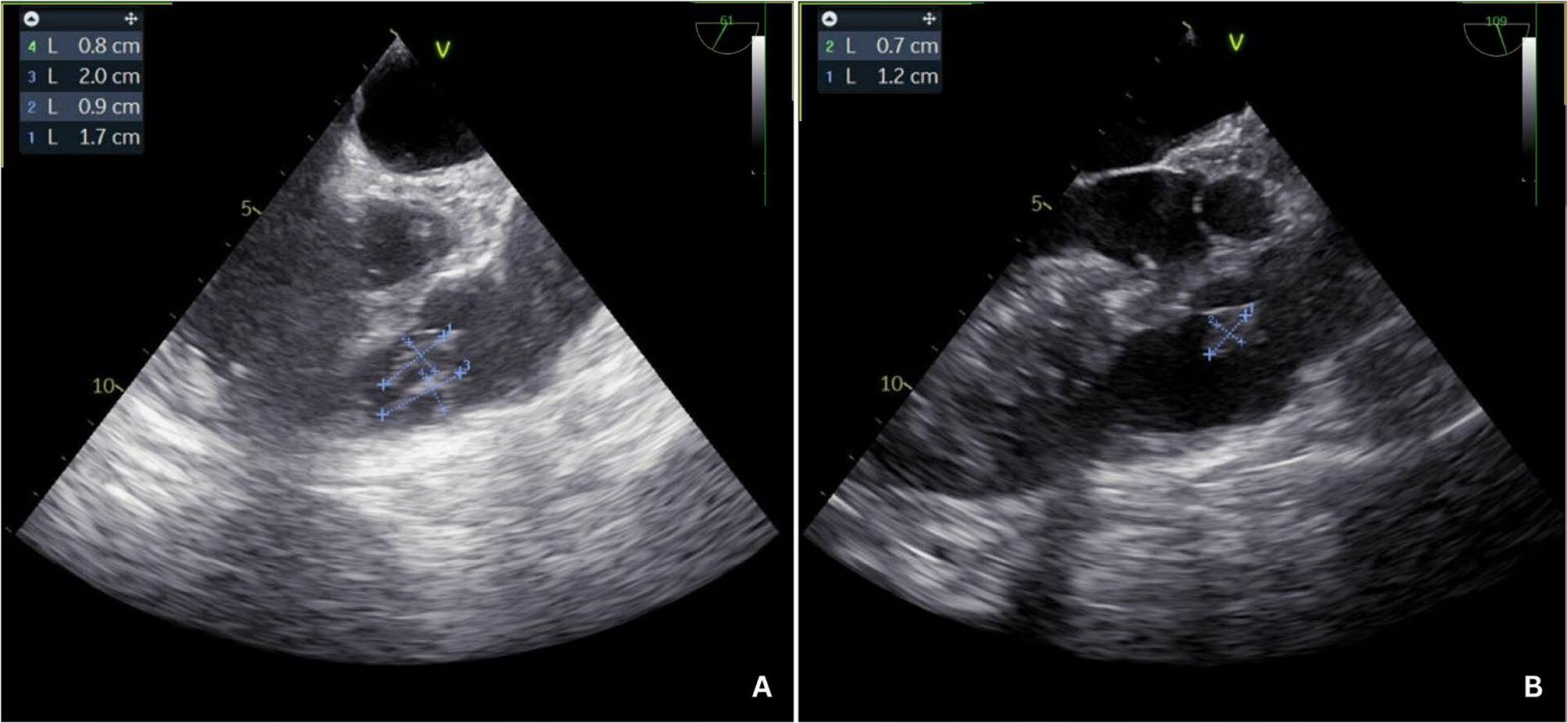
Transesophageal echocardiogram (TEE). A. Pre-procedural TEE. 61º. Two intracardiac masses suggestive of vegetations adhered to the pulmonary valve measuring 2.0 x 0.8 cm and 1.7 x 0.9 cm. B. Post-procedural TEE. 109º. One residual vegetation adhered to the pulmonary valve measuring 1.2 x 0.7 cm
